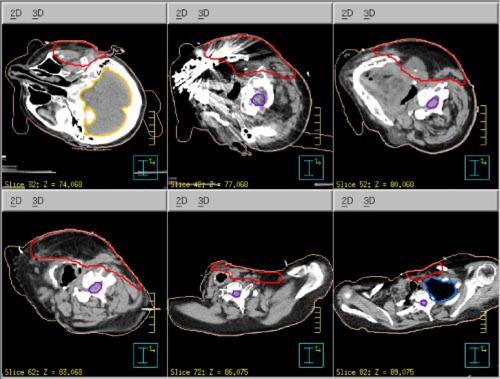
(Color) Transverse CT slices of Patient 2 showing the surface anatomical defect and the location, depth, and shape of the parotid PTV at various levels.
